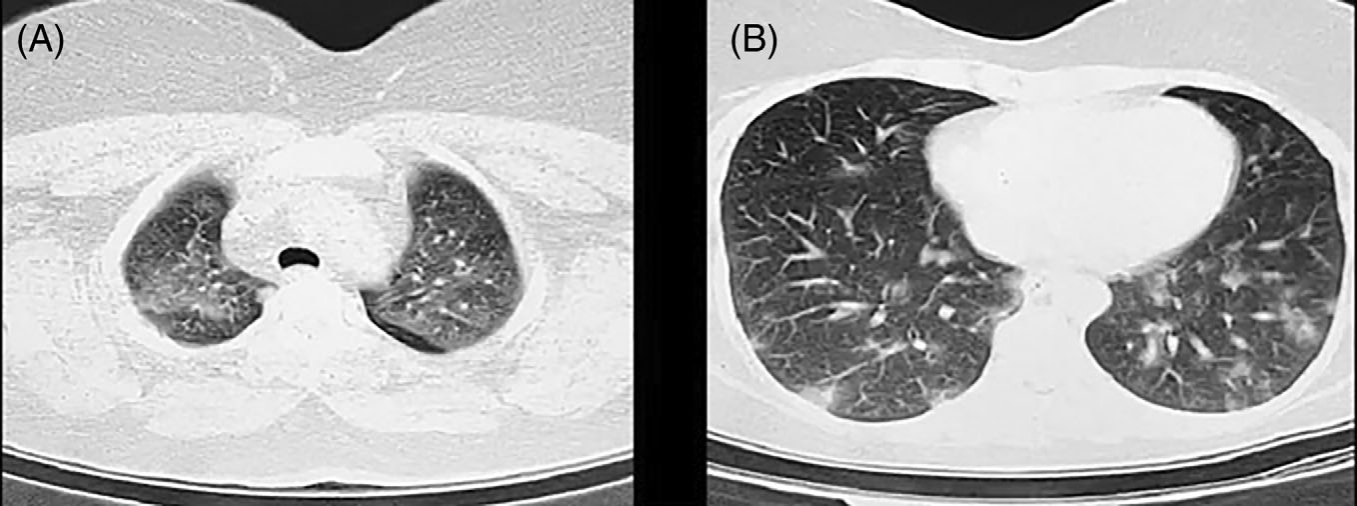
An axial section of the chest computed tomography [CT] scan. Ground glass opacities (A) and peripheral consolidation (B)
